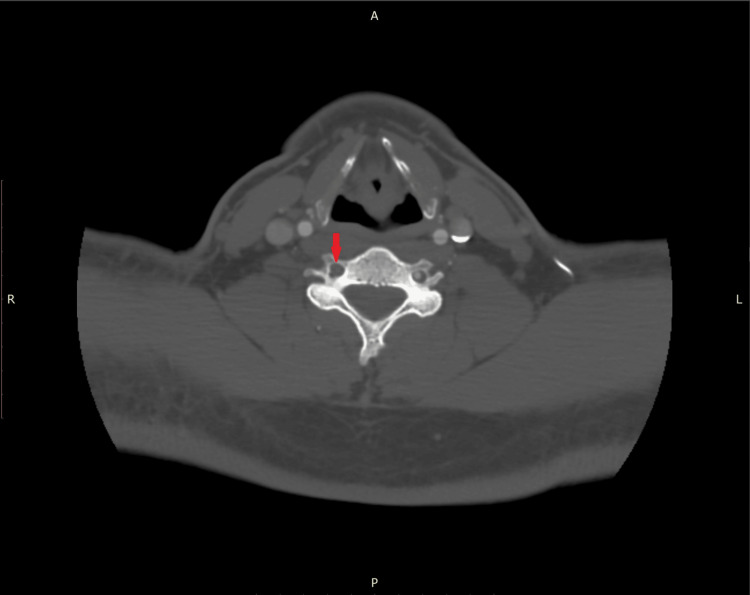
Repeat CT angiography obtained six weeks from presentation demonstrating persistent non-opacification (red arrow) of the right vertebral artery.
